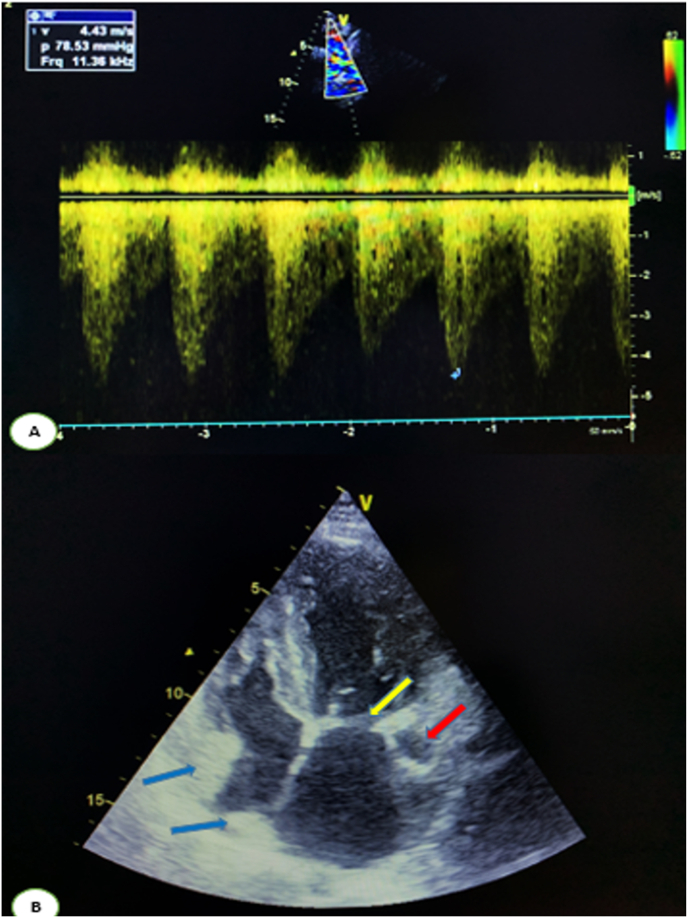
(A) Echocardiographic suprasternal view showed the presence of coarctation of the aorta with V max at 4.43 m/s with a mean gradient at 79 mmHg and an extended speed reduction during diastole. (B) Apical 4-chamber view, demonstrating sub-mitral membrane (yellow arrow), with a single sub-papillary muscle, two non-motile rounded hyper-echogenic formations in the right atrium (blue arrow), suggest embryonic residue, with a dilated coronary sinus (red arrow). (For interpretation of the references to colour in this figure legend, the reader is referred to the Web version of this article.)
